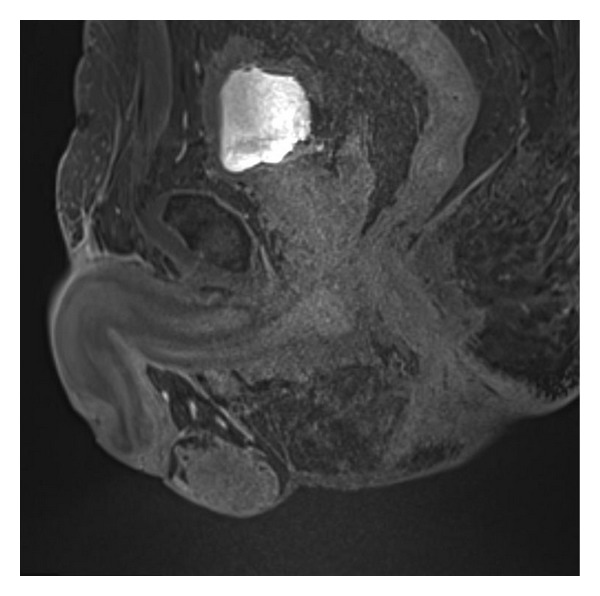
MRI scan which shows a focal lesion on the dorsal side of the glans penis.
